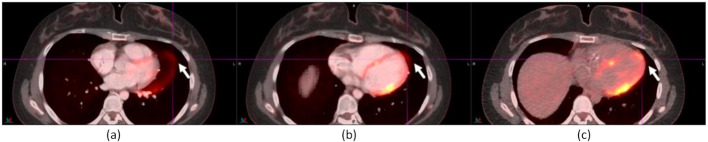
The anterior-lateral walls (indicated by an arrow) of the heart were registered in DDG CT (c), mostly registered in AIR (b), but significantly misregistered in baseline (a)
